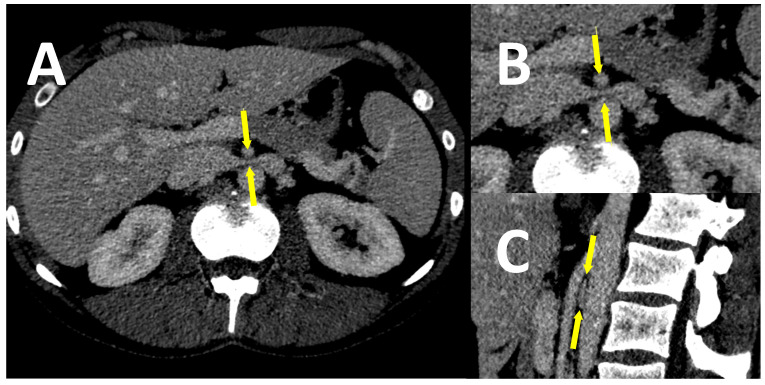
The images of a CT-V scan in a patient with LRV entrapment (“nutcracker” syndrome). LRV (marked with arrows) is compressed between the aorta and superior mesenteric artery (SMA), as shown on the transverse plane—whole image (A), enlarged image (B), and on the sagittal plane (C).
